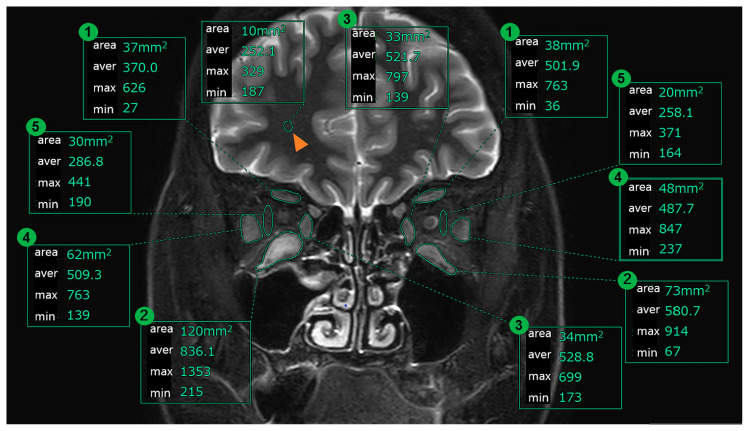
Coronal MRI and measurement method. Note—area: cross-sectional area (mm^2^), aver: average of STIR signal intensity, max: maximum of the STIR signal intensity within the selection, min: minimum of the STIR signal intensity within the selection, orange arrows: white matter, 1: SRM, 2: IRM, 3: MRM, 4: LRM, and 5: orbit fatty tissue.
